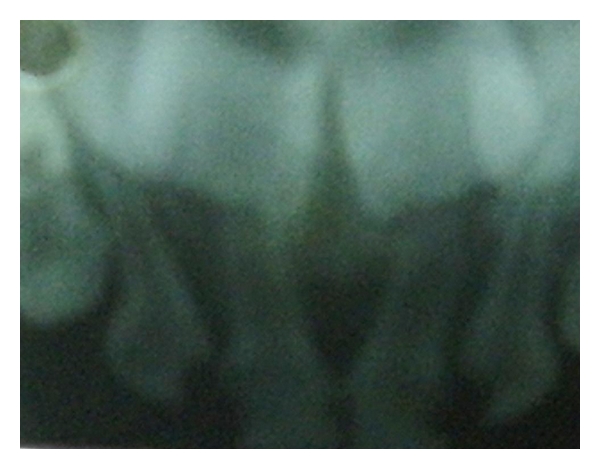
Intraoral periapical radiographs revealed pulp involvement of 52, 51, 61, and 62.
